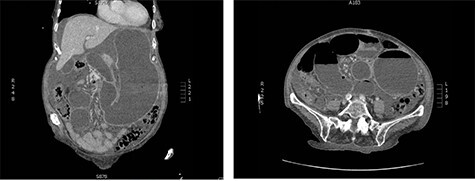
CT abdomen and pelvis on presentation; swirling of the SMA, markedly distended stomach and small intestine are noted.
